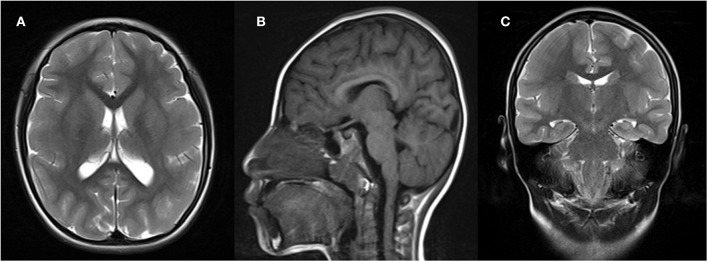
Brain magnetic resonance imaging (MRI) of the patient, showing microcephaly and simplified gyrus of the cerebral cortex. (A) Axial T1-weighted image; (B) sagittal T1-weighted image; (C) coronal T1-weighted image.
